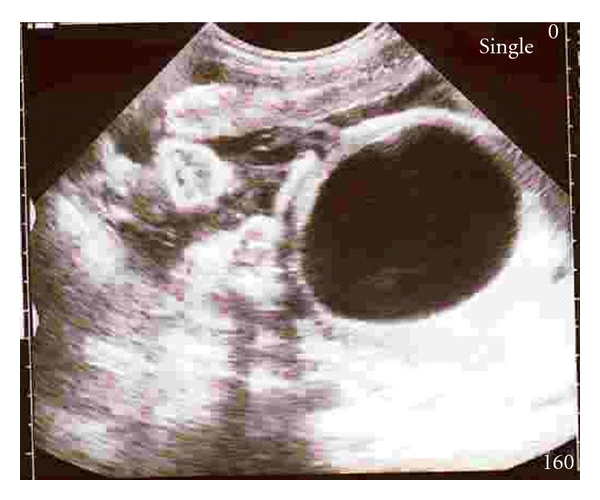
Prenatal ultrasound picture showing intra-abdominal cyst measuring 8 × 8 cm.
